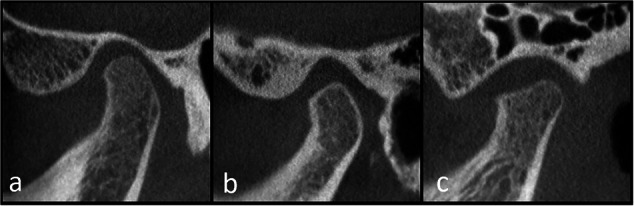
Cone-beam CT, sagittal view of the TMJ, (a) a 12-year-old boy with a normally shaped fossa-eminence and condyle, (b) a 14-year-old girl with a normal fossa, but subtle anterior flattening of the condyle, and (c) a 15-year-old with a moderately widened fossa-eminence and a mildly to moderately flattened condyle. This figure has been reproduced from [46] under the Creative Commons Attribution 4.0 International License
